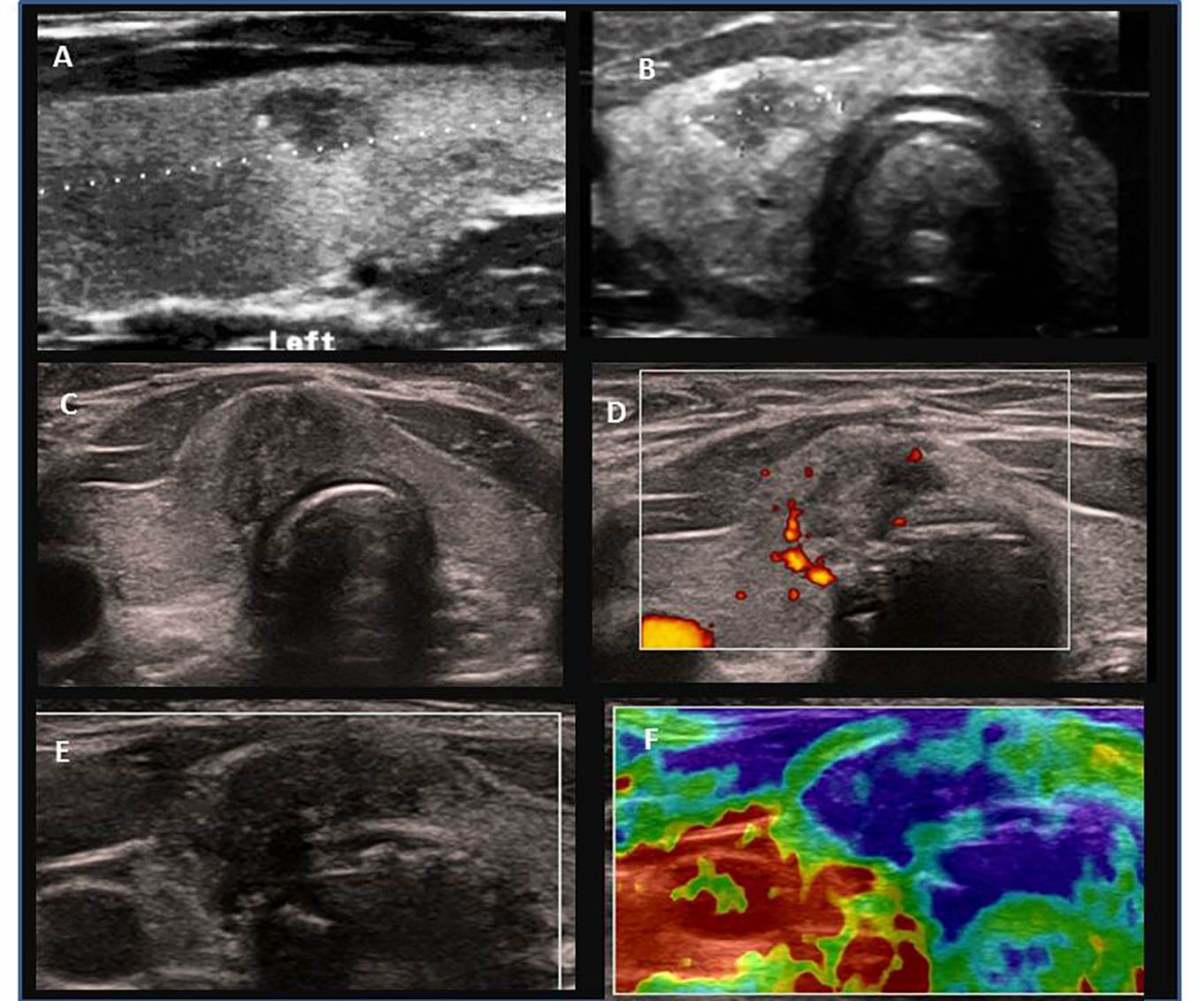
Ultrasound presentation of thyroid nodules confirmed as PTC in patients AIT (-). (A) female, 11.9 years old, multifocal (M), bilateral (B), PTC 7.6 mm, classic; (B) male, 15 years, M, B, PTC 9.5 mm, classic; (C–F) female, 14 years old, M,B, PTC 20 mm, classic, follicular, solid; (D) increased peripheral vascularization, (F) elastography presented that malignant lesion was stiffer (blue colour) than surrounding thyroid tissue (green, yellow, red colour).
